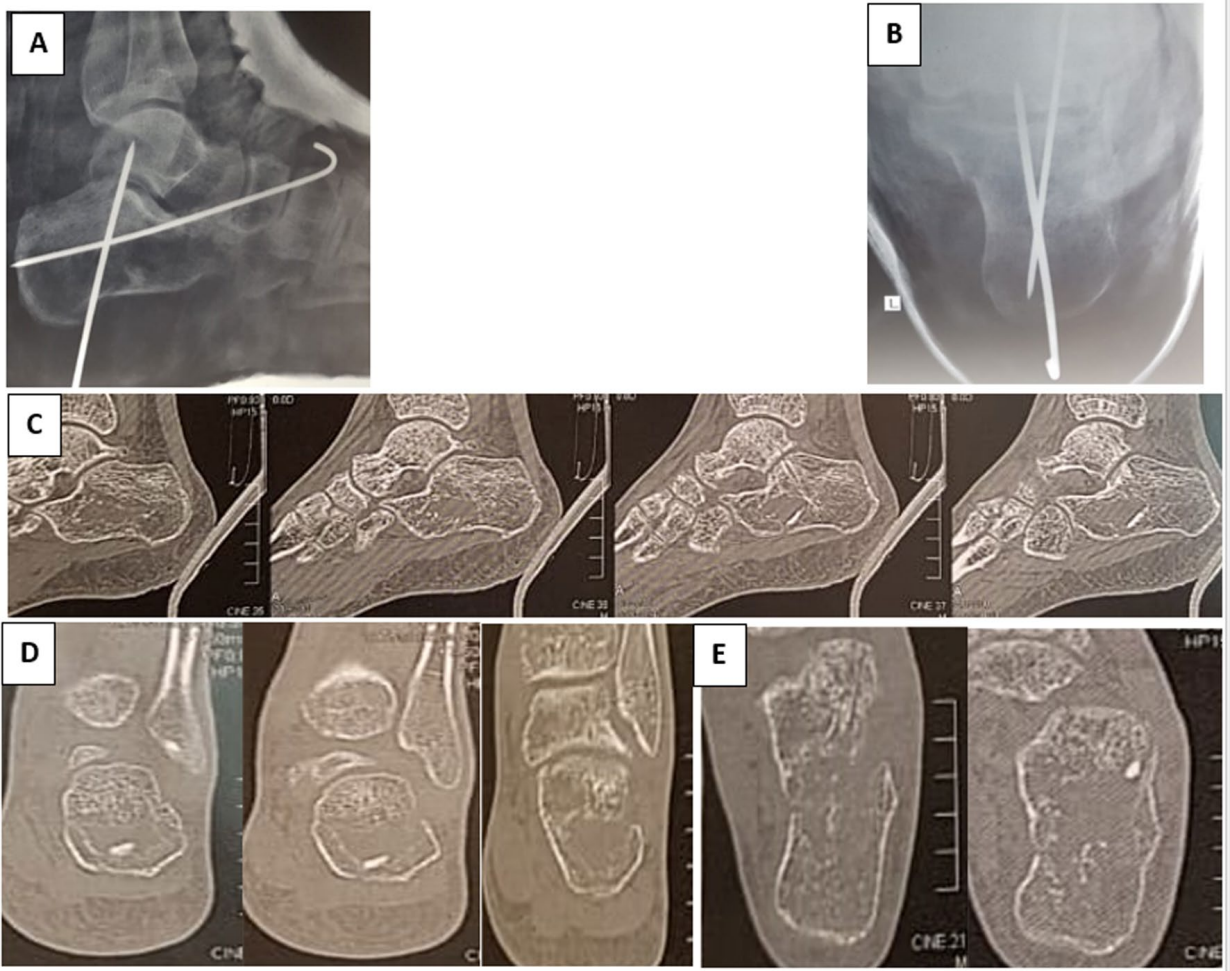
A, B Immediate postoperative lateral and axial plain X-rays, respectively. C, D, E 6 months postoperative CT
